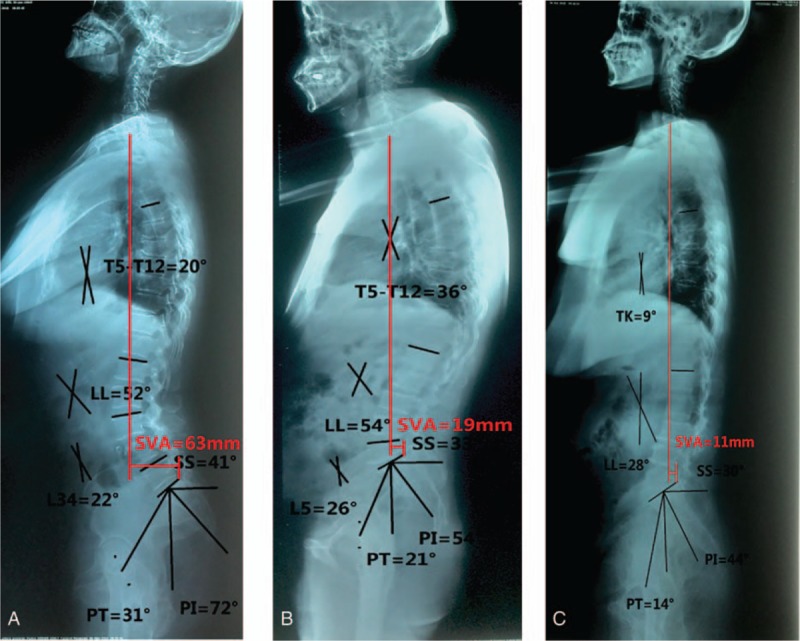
(A) A male, 47-year old. The lateral full spine X-ray shows L3 to L4 spondylolisthesis. PI = 72°, PT = 31°, SS = 41°, LL = 52°, T5 to 12 = 20°, CSL = 22°. (B) A male, 49-year old. A lateral full spine X-ray shows L5 spondylolisthesis. PI = 54°, PT = 21°, SS = 33°, LL = 54°, T5 to 12 = 36°, CSL = 26°. (C) A male, 48-year old. A lateral full spine X-ray shows no lumbar spondylolisthesis. PI = 44°, PT = 14°, SS = 30°, LL = 28°, T5 to 12 = 9. CSL = Cobb angle of spondylolisthesis level, LL = lumbar lordosis, PI = pelvic incidence, PT = pelvic tilt, SS = sacral slope, SVA = sagittal vertical axis, T5 to T12 = Cobb from the fifth thoracic vertebral to 12th thoracic vertebral.
